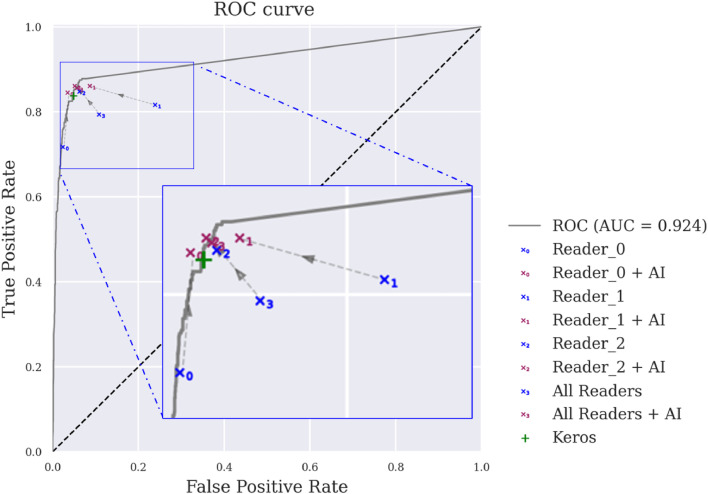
Results of a retrospective study to evaluate the impact of AI assistance on the diagnostic accuracy of radiologists for detecting bone marrow edema in knee MRI: Receiver operating characteristics (ROC) curve comparing generalist radiologist performances without and with AI aid and Stand Alone AI (Keros). AUC: area under the curve; AI: artificial intelligence.
